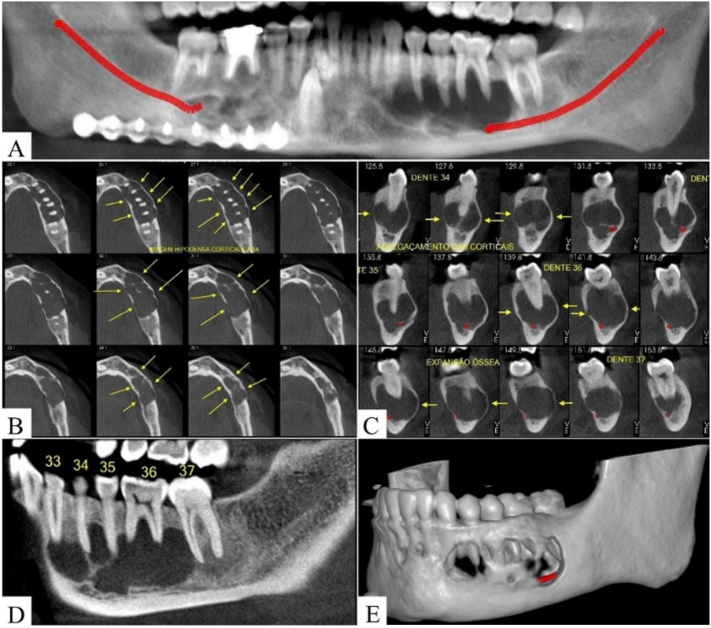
Initial CT imaging analysis. (A) CT in panoramic reconstruction shows single, extensive and mixed lesion in left mandibular region, closer to inferior alveolar nerve (red line). Axial (B) and sagittal (C) sections showed bulging and thinning of buccal and lingual plates (yellow arrows). (D) Lateral view and (E) 3D mandibular reconstruction showed extensive volume of FD.
